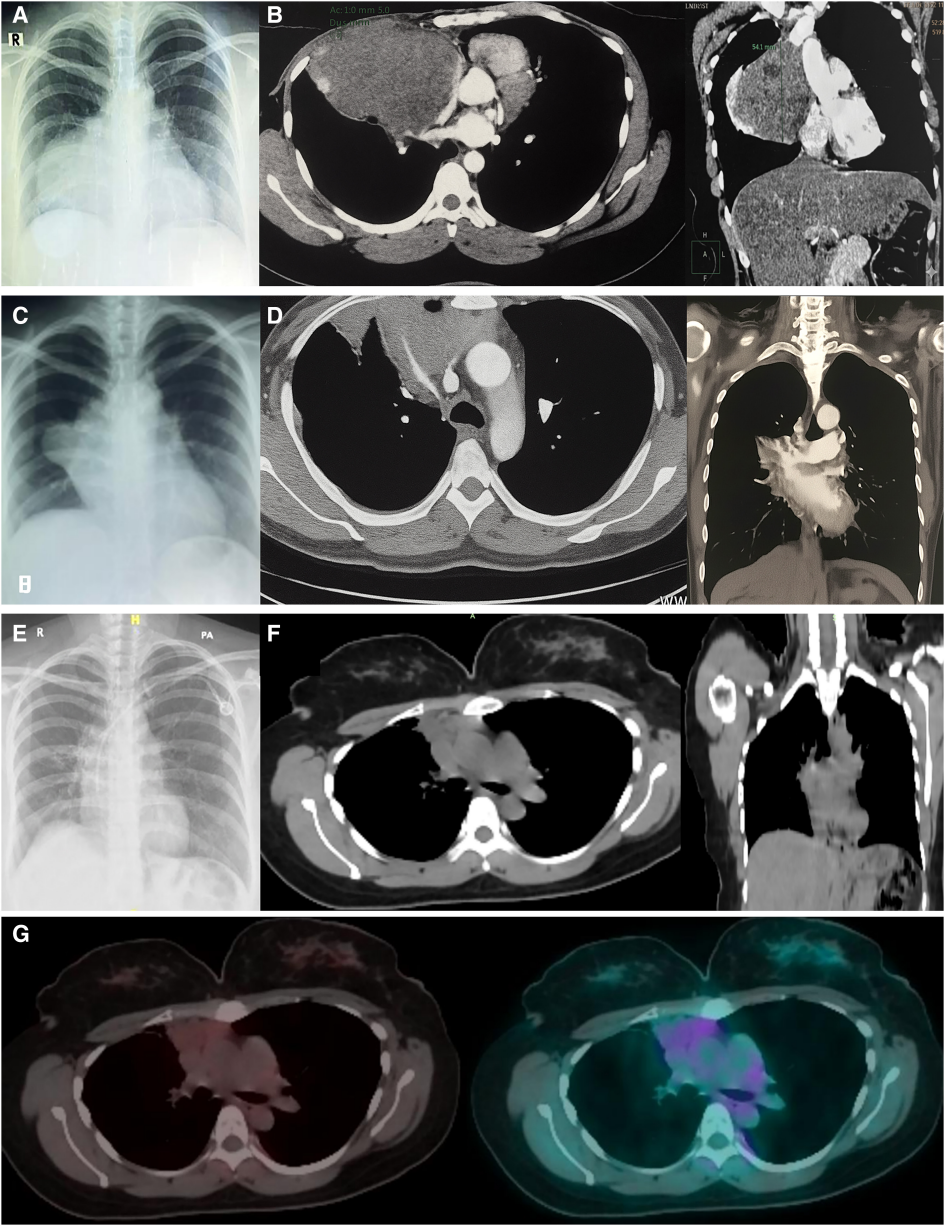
Chest imaging. (A) Pre‐treatment chest x‐ray (May 2022) showing mediastinal mass (9.8 × 7.2 × 8.1 cm); (B) pre‐treatment CT revealing a large anterior mediastinal mass (9.8 × 7.2 × 8.1 cm) compressing the heart and SVC; (C) post‐resection chest x‐ray at one‐month follow‐up showed marked reduction of the mediastinal mass (7.5 × 4.8 × 7.7 cm); (D) post‐resection CT showing residual mass (7.5 × 4.8 × 7.7 cm) with pericardial lesion; (E) chest x‐ray showing a thymoma mass measuring 2.2 × 1.1 × 1.3 cm after three‐month follow‐up completion of multimodal treatment, including surgical resection, radiotherapy, and pembrolizumab; (F) CT scan showing a residual lesion measuring 2.3 × 1.0 × 1.3 cm with no evidence of new mass formation after three‐month follow‐up completion of multimodal treatment; (G) post‐completed immunotherapy PET scan at six‐month follow‐up revealed an irregular‐margined (2.2 × 1.1 × 1.3 cm), likely representing non‐viable tumour residue. No lesions are seen in the lymph nodes or other organs suspicious for additional malignancy.
